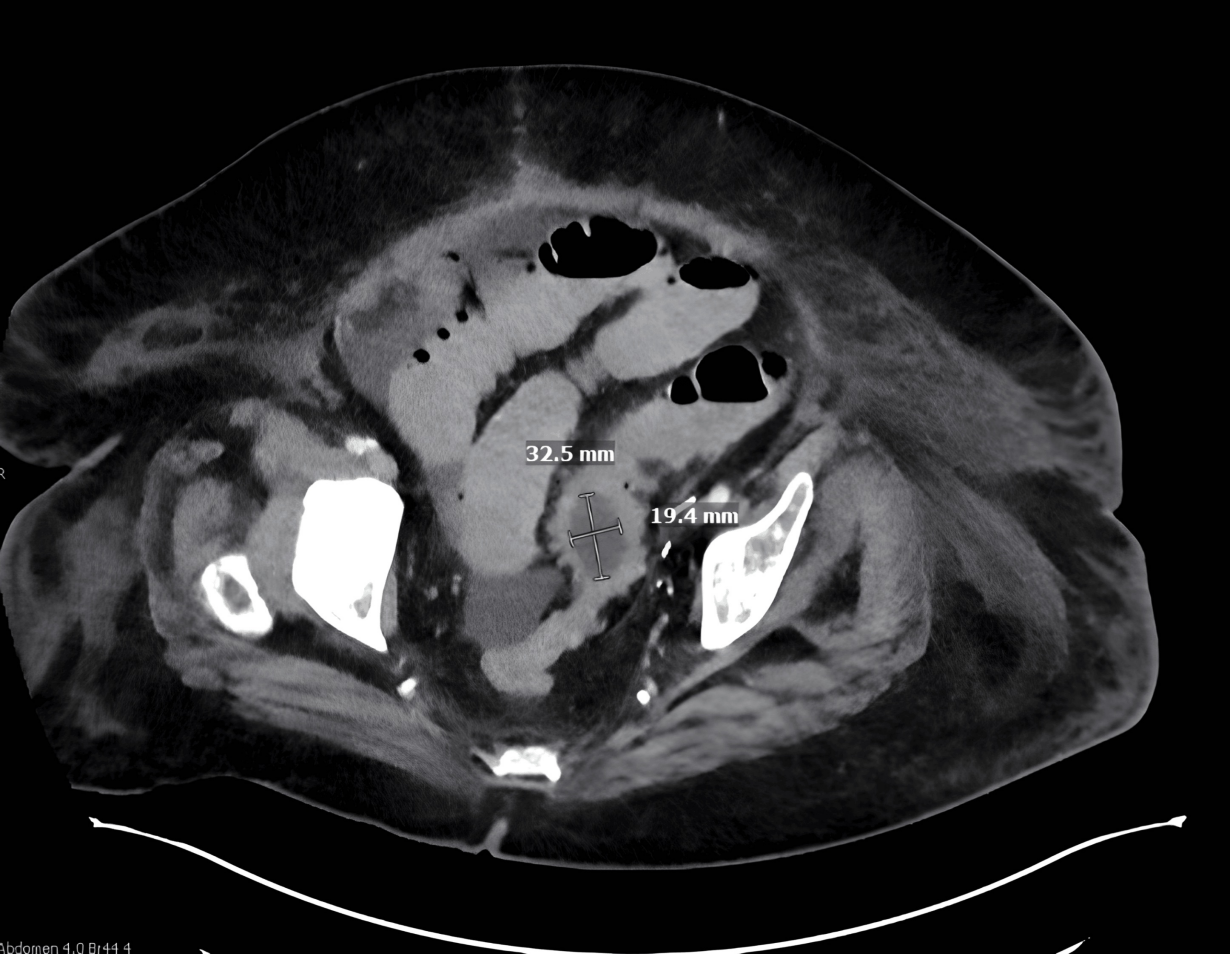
Abdominal CT gallstone mistaken for fecalith/stool.
